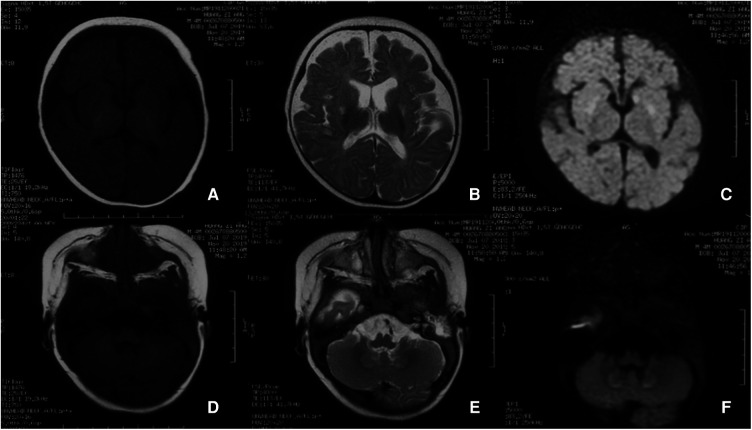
Brain MRI of case 2 at the beginning of the disease. Presence of roughly symmetrical abnormal signal foci in the head of the caudate nuclei, lenticular nuclei, and dentate nuclei of the cerebellar hemisphere.
